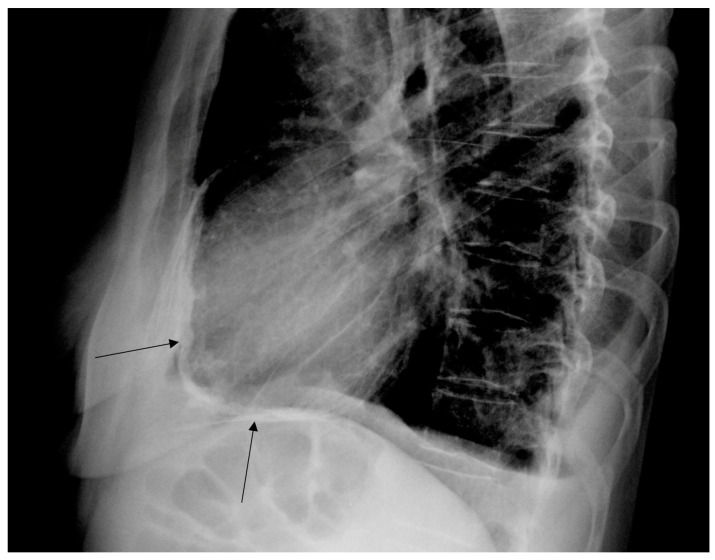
Constrictive pericarditis (arrows) (lateral chest X-ray).
